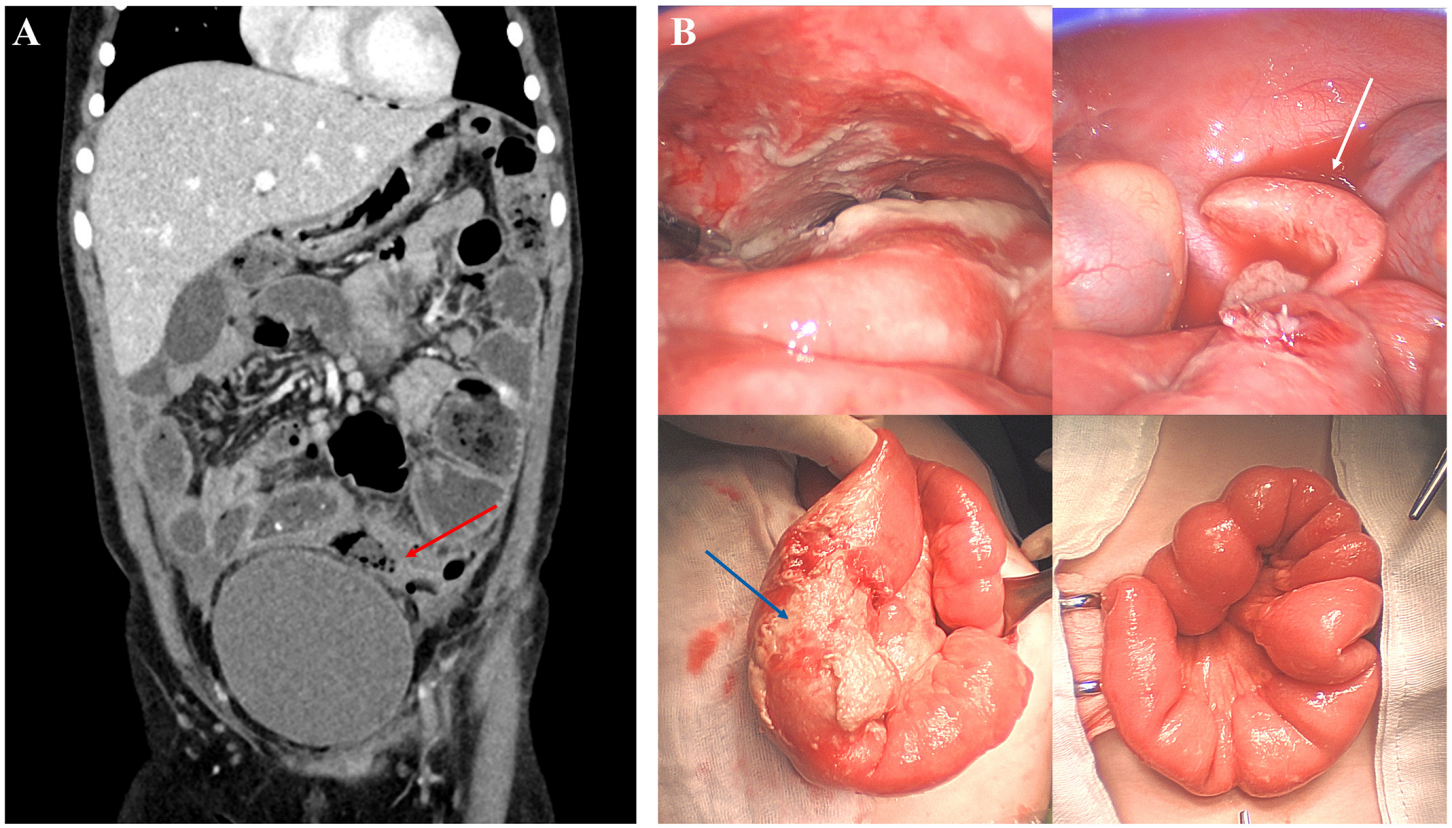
Imagings before and during 2nd surgery. (A) CT scan showing intraperitoneal free air and multifocal abscess in pelvic cavity, suggesting bowel perforation. Possible perforation site was sigmoid colon or jejunoileal junction (red arrow), but not definite. (B) Intraoperative images of pelvic inflammation and abscess. Appendix was swollen (white arrow), and suspected sealed-off perforation site was reinforced (blue arrow). Ring-like strictures were still noted as before.
